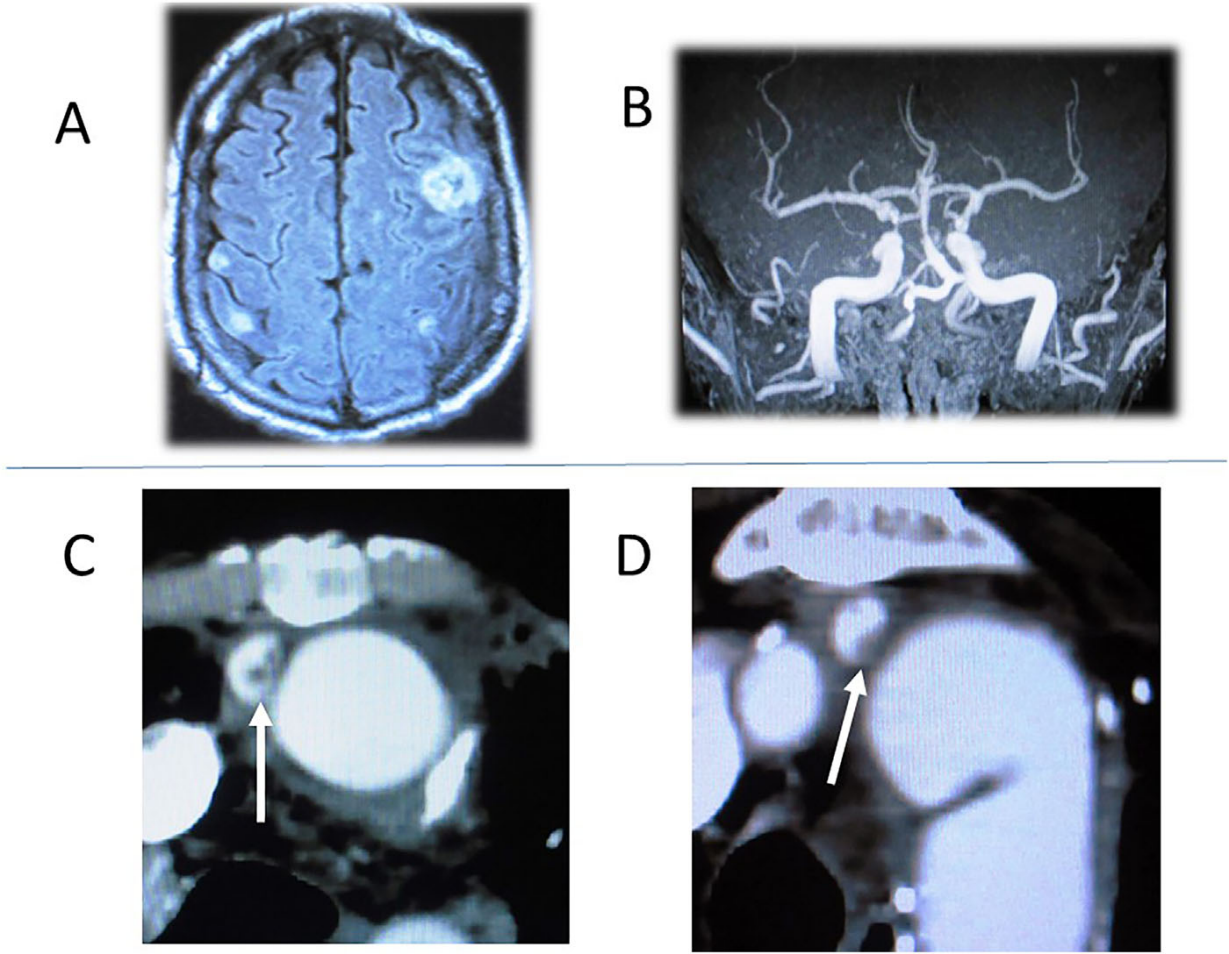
Preoperative magnetic resonance image and enhanced computed tomography. A, Multiple fresh mycotic brain infarctions were detected. B, Severe stenosis of the right internal carotid artery was detected. C, Vegetation was detected in the brachiocephalic graft branch. D, Vegetation was detected in the left common carotid branch
